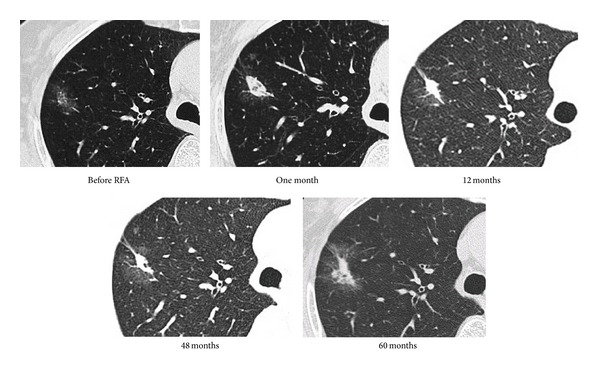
Serial CT findings before and after initial bronchoscopy-guided cooled radiofrequency ablation (RFA) in case 1.
